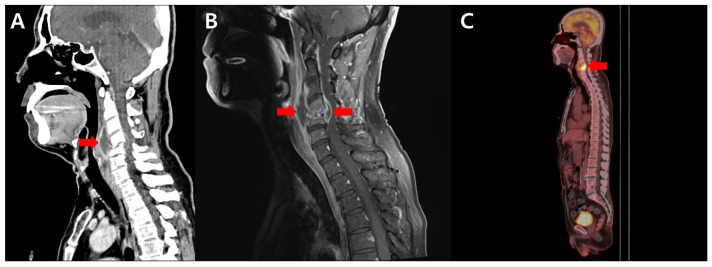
Follow-up preoperative progression and diagnostic confirmation. (A) Enhanced neck CT confirming an ~4 cm hypo-attenuated prevertebral abscess with rim enhancement at the C3–5 level, causing severe cord compression (red arrow). (B) T1-weighted fat-suppressed sagittal cervical spine MRI showing significant progression with enlarged prevertebral and epidural abscesses (red arrows), increased bone marrow infiltration, and a C5 compression fracture, despite the ongoing cephalexin therapy. (C) PET-CT showing diffuse FDG uptake in the C4–5 vertebral bodies and prevertebral space, with a compression fracture at the C5 level (red arrow).
